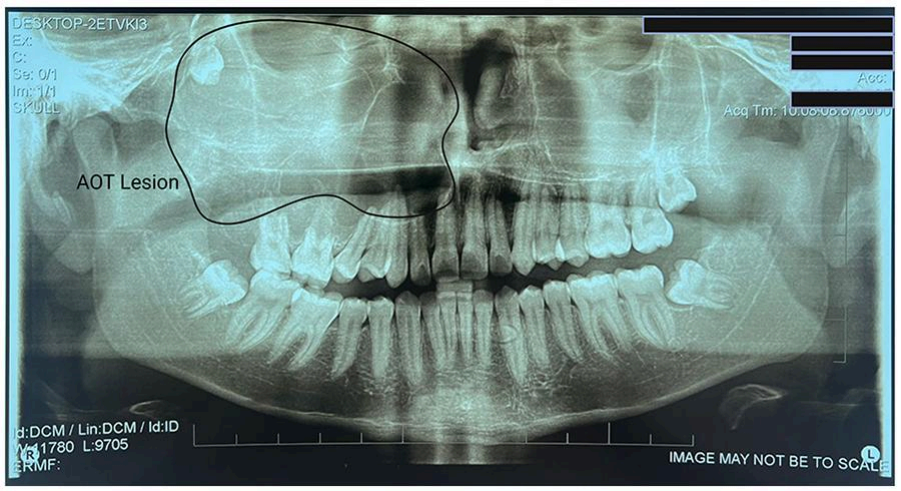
Preoperative panoramic radiograph of the maxilla and mandible. Radiograph showing a multilocular radiolucent lesion with internal calcifications in the right hemimaxilla, involving teeth 1.5, 1.6, 1.7, and 1.8. The lesion causes displacement of the third molar (1.8) toward the floor of the right orbit, which remains intact. It exhibits a “soap bubble” appearance and well-defined borders.
